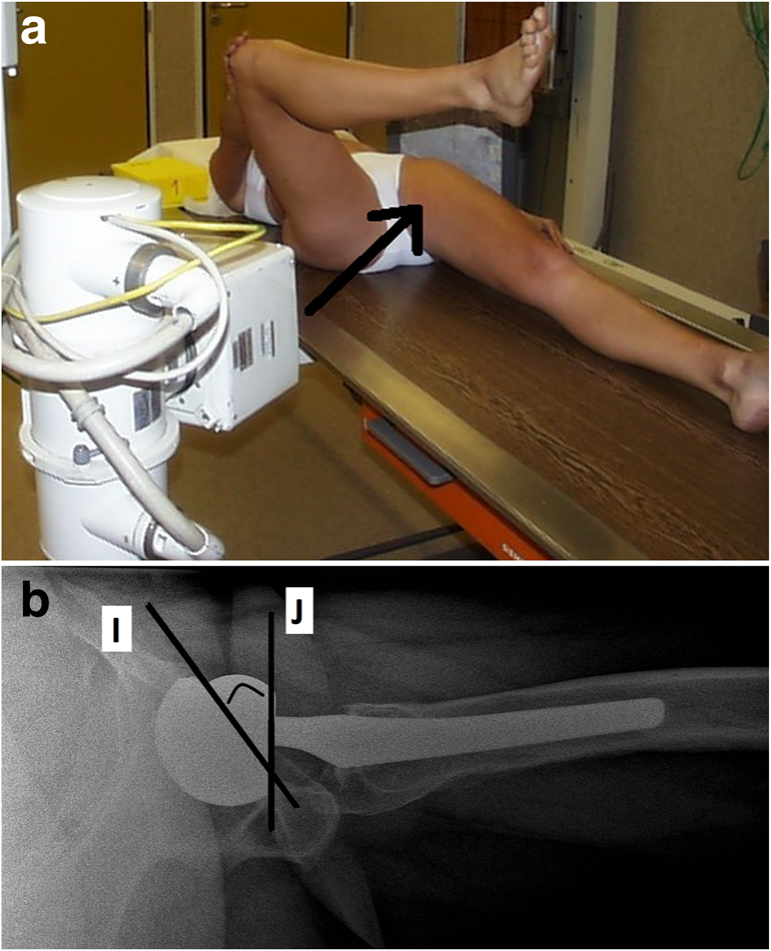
a Photograph of the patient positioning for a lateral view of the hip, the *arrow* indicating the direction of the x-rays. b Lateral radiograph. The acetabular anteversion is defined by the angle between the acetabular axis (line *I*) and the coronal plane (line *J*). In this patient, the angle measures approximately 25° (normal range between 5°–25°)
